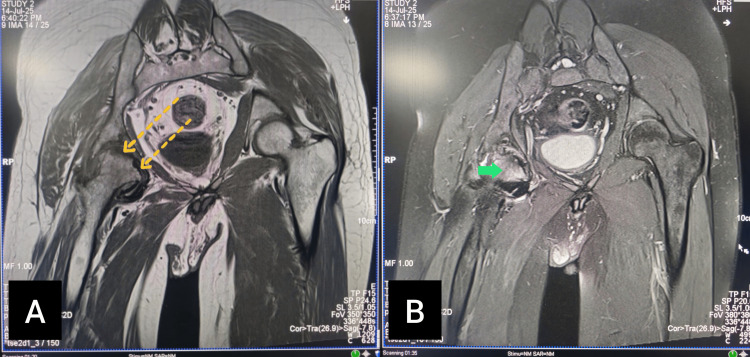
MRI of the right hip joint (A) Coronal T1 sequence reveals hypointensities in the subchondral region of the right femoral head (dotted yellow arrows). (B) Proton density fat-saturated (PDFS) sequence shows subchondral hyperintensities in the right femoral head (solid green arrow) MRI: magnetic resonance imaging
